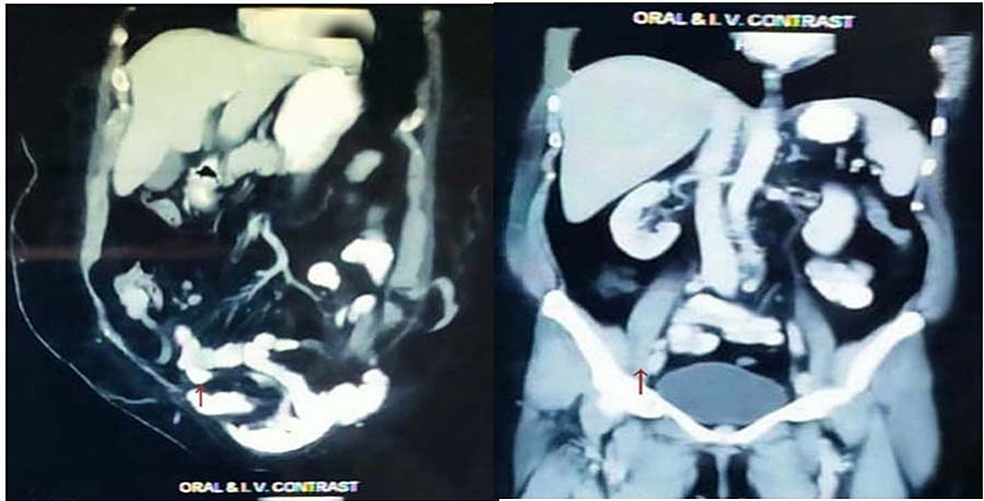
Follow-up computed tomography in sagittal and coronal view of the whole abdomen. CT showing the hyperdense area in colostomy site and vaginal stump suggestive of metastatic deposits (arrows), rest of the abdominal organs were normal.
